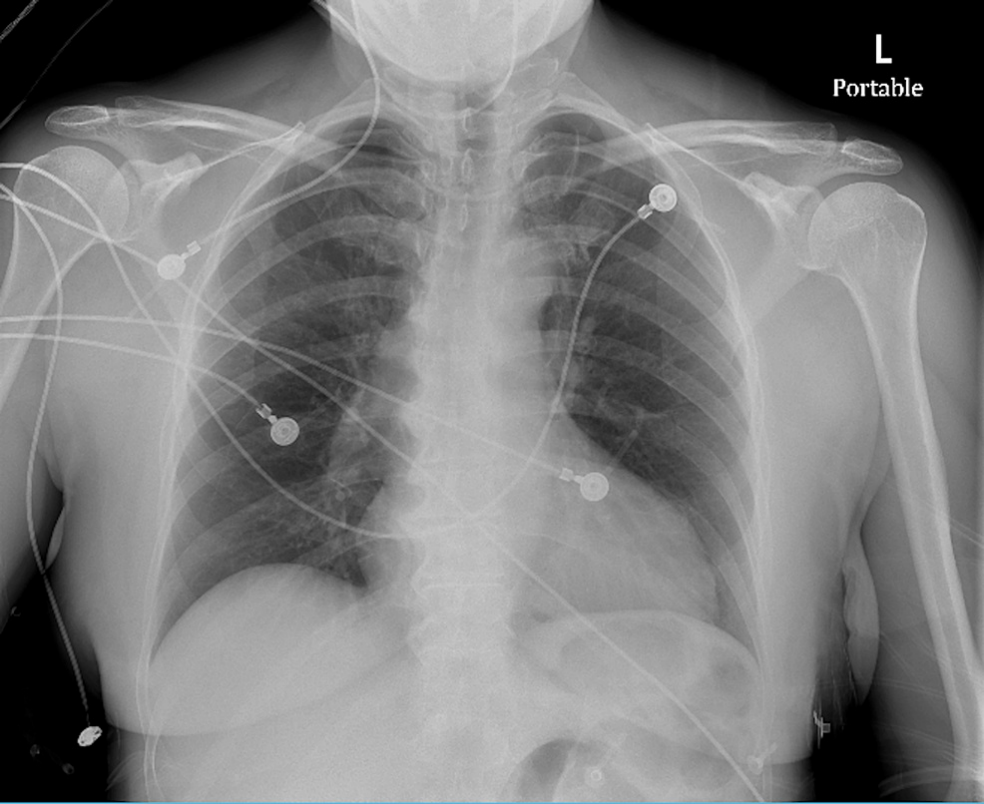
Anteroposterior chest radiograph demonstrating no acute intrathoracic pathology
